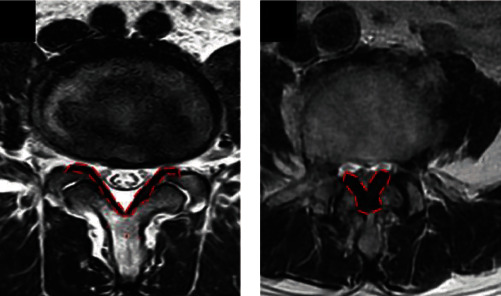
MRI scans of the lumbar spine. T2-weighted MRI scans of the LF (surrounded by orange broken lines) of (a) a patient with LDH and (b) a patient with LSS.
